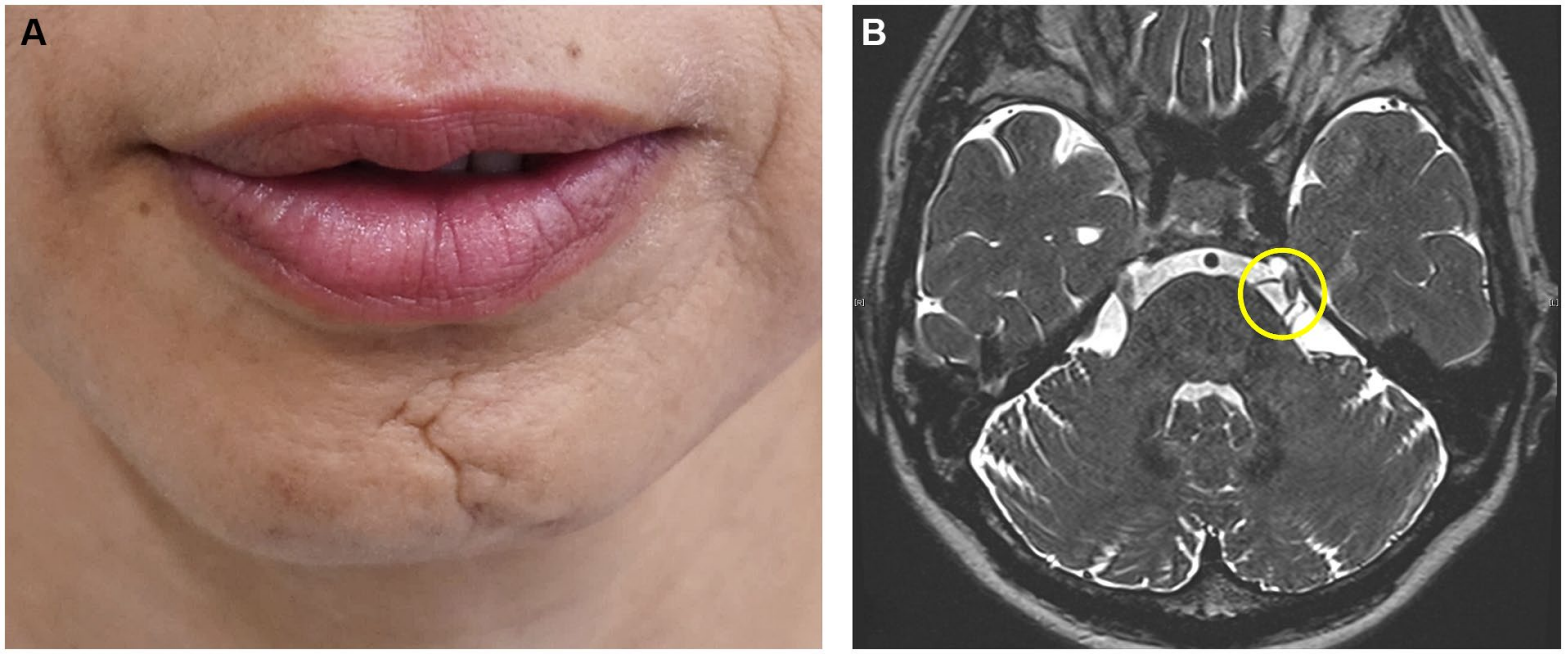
Characteristic clinical features of hemimasticatory spasm Linear scleroderma of the chin (A). Compression of the left trigeminal nerve by an artery is suspected on magnetic resonance imaging (B).
